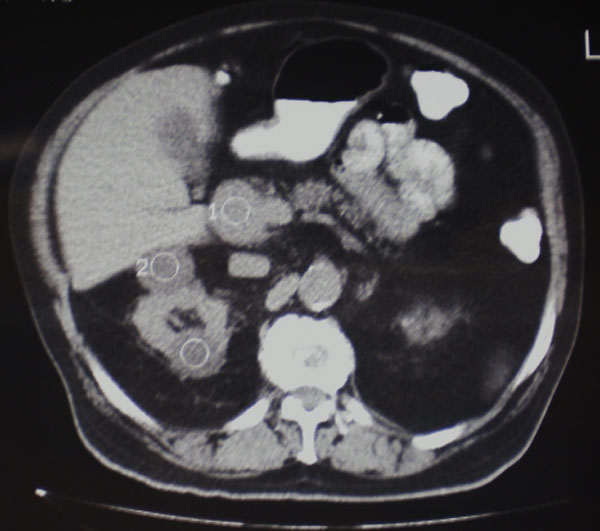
CT scan image showing a large mass of 6.8 cm in diameter in direct contact with the head of the pancreas and the inferior vena cava (marked as 1 on the figure - marked as 2 & 3 coexisting simple kidney cysts).
